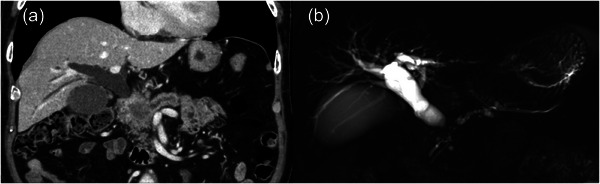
Contrast‐enhanced computed tomography (CE‐CT) and magnetic resonance cholangiopancreatography (MRCP) images at diagnosis. (a) CE‐CT image showing a pancreatic head tumor and dilation of the main pancreatic duct on the caudal side. The distal bile duct is obstructed by the pancreatic head tumor. It was considered borderline resectable according to the NCCN clinical practice guideline version 2.2021. (b) MRCP image showing dilatation of the main pancreatic duct distal to the pancreatic head tumor. The intrahepatic bile ducts were dilated up to 7 mm, and the extrahepatic bile duct was dilated up to 14 mm.
